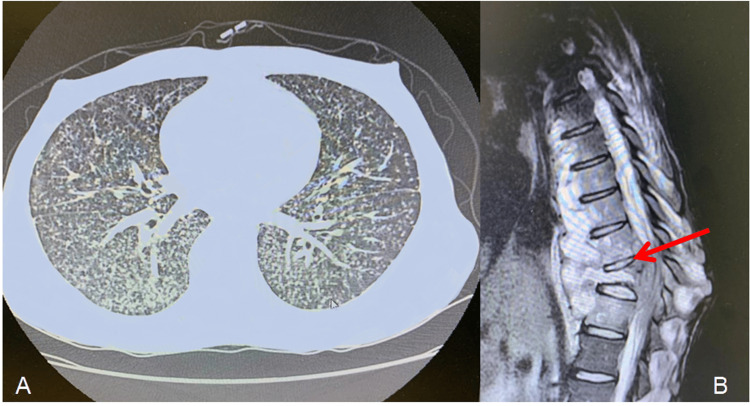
Patient's CT scan and MRI A. CT scan without intravenous contrast showing bilateral, diffuse, micronodular pulmonary involvement compatible with miliary TB. B. The MRI study showing the severe vertebral inflammatory process with vertebral collapse and paravertebral contrast enhancement (red arrow), compatible with Pott’s disease.
